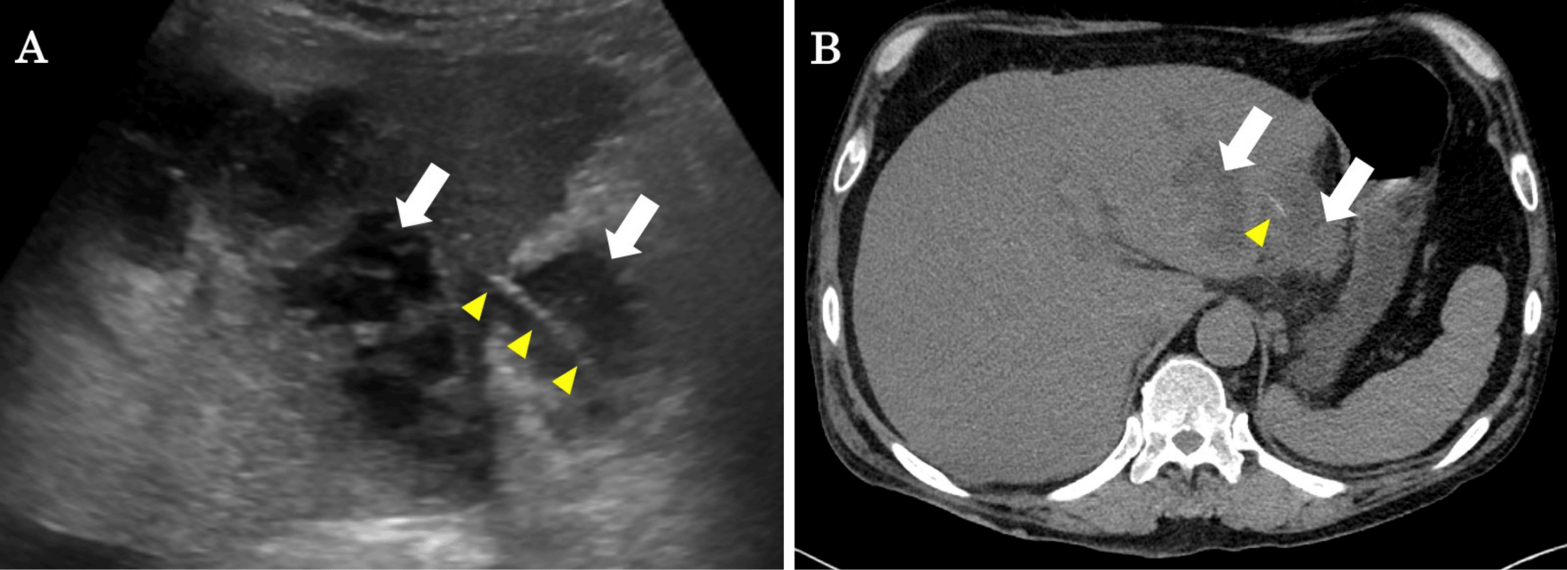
Abdominal ultrasound and CT findings. (A) There are lesions suspected to be abscesses in the left lobe of the liver and adjacent to the liver (white arrows). A linear hyperechoic lesion was observed penetrating from the extrahepatic mass into the liver (yellow arrows). (B) Low attenuation area lesions suspected to be abscesses (white arrows) and linear high attenuation area lesions suspected to be foreign bodies (yellow arrow) were found. CT: computed tomography
